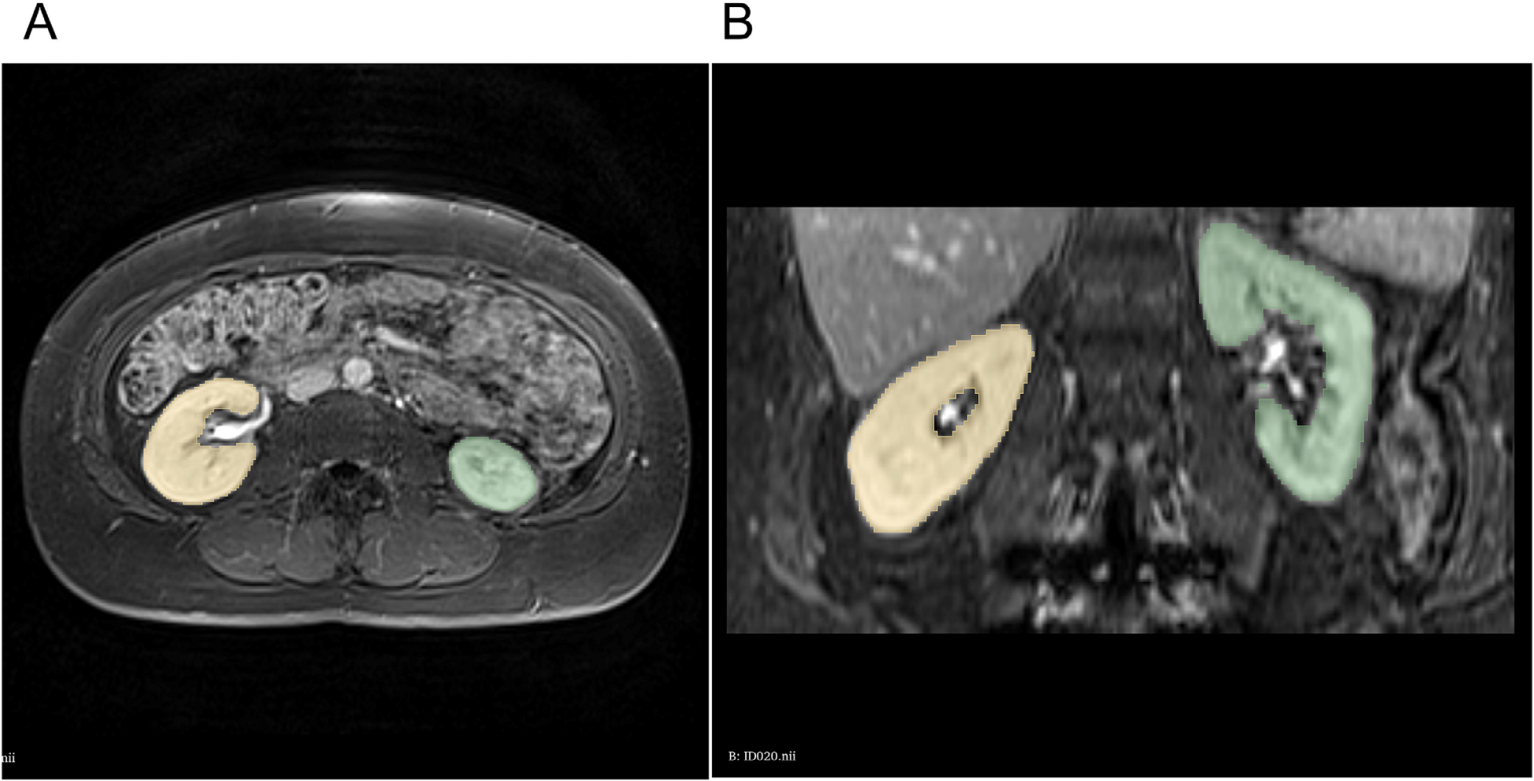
MRI T1‐VIBE axial (A) and coronal (B) images with labeled left (green) and right (yellow) kidney.
